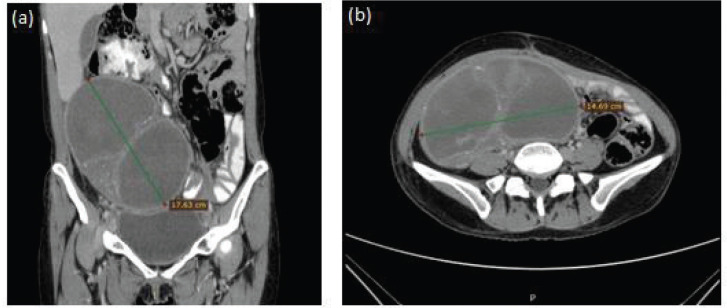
Representative images of contrast-enhanced abdominal CT scan showing partial tumour response. (a) Coronal section with 17.63 cm tumour (green line) and without ascites. (b) Axial section with 14.69 cm tumour (green line).
